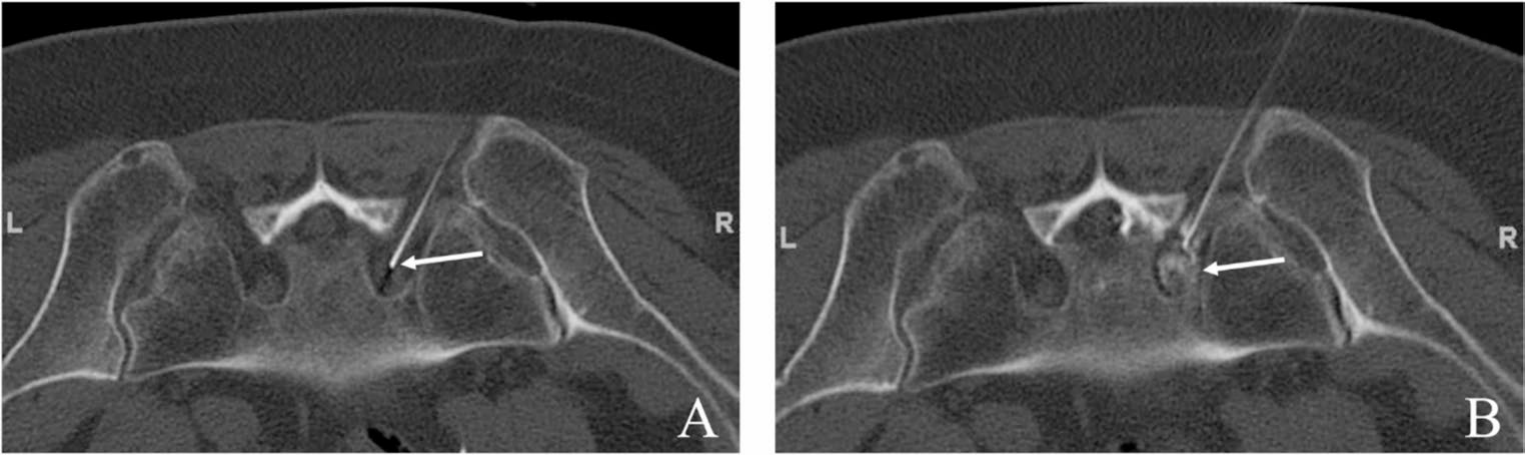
Typical example of CT-guided periradicular infiltration of the S1 nerve root on the right hand side of a 74-year-old female patient, performed in prone position. (A) final needle position before drug administration, focusing on the slice where the needle tip (white arrow) is positioned directly adjacent to the S1 spinal nerve in the neuroforamen. (B) final CT-documentation to prove proper periradicular distribution of the drug cocktail (local anesthetics and cortical steroids mixed with iodinated contrast medium, white arrow)
